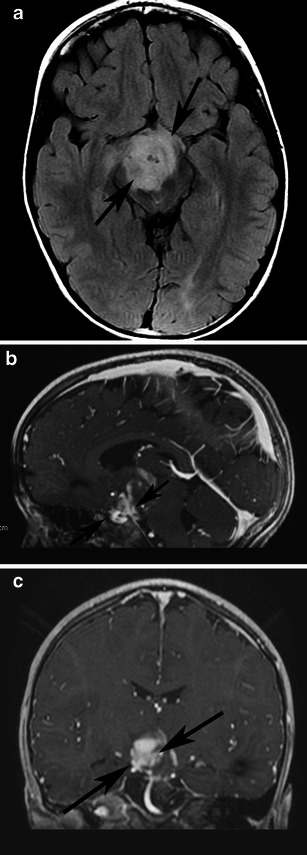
Pilomyxoid astrocytoma in a 5-year-old girl. a An axial FLAIR image shows a large solid lobulated suprasellar mass with uniform hyperintensity (arrows). Postgadolinium sagittal (b) and coronal (c) T1-weighted images reveal a homogeneously enhancing suprasellar infiltrative tumour (arrows)
